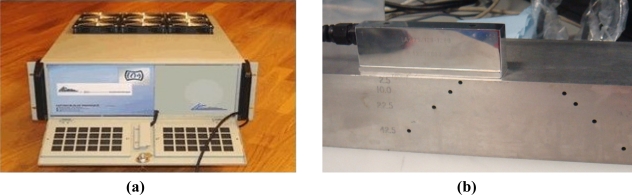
Experimental apparatus. (a) OPEN ultrasound phased array control system (LeCouer, France), (b) 5 MHz, 128-element linear transducer array (Vermon, France) and test sample of solid steel block with multiple side-drilled holes. Transducer array is in contact with sample upper surface with gel coupling.
